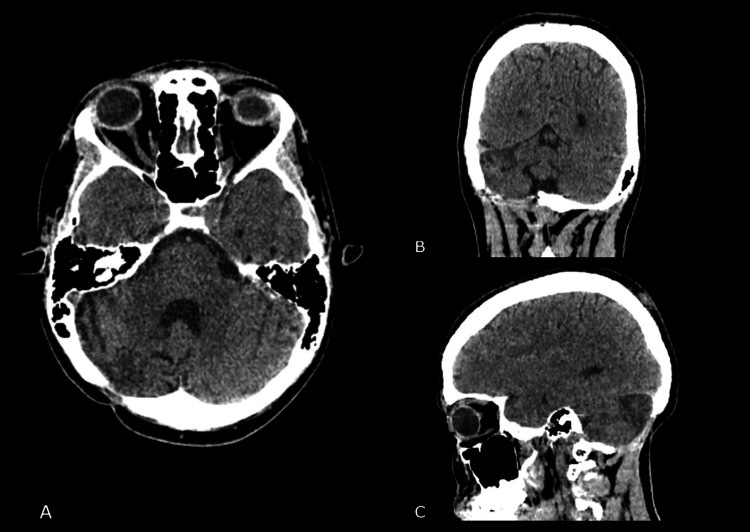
Follow-up computed tomography scan one year after surgery (A: axial; B: coronal; C: sagittal).
